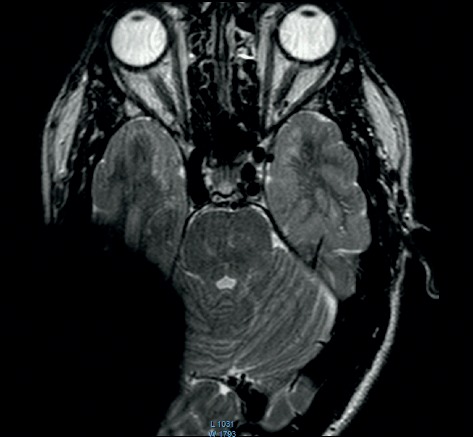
MRI pattern of the axial plain of a 1.5 T scanning Medel Synchrony Implant.
